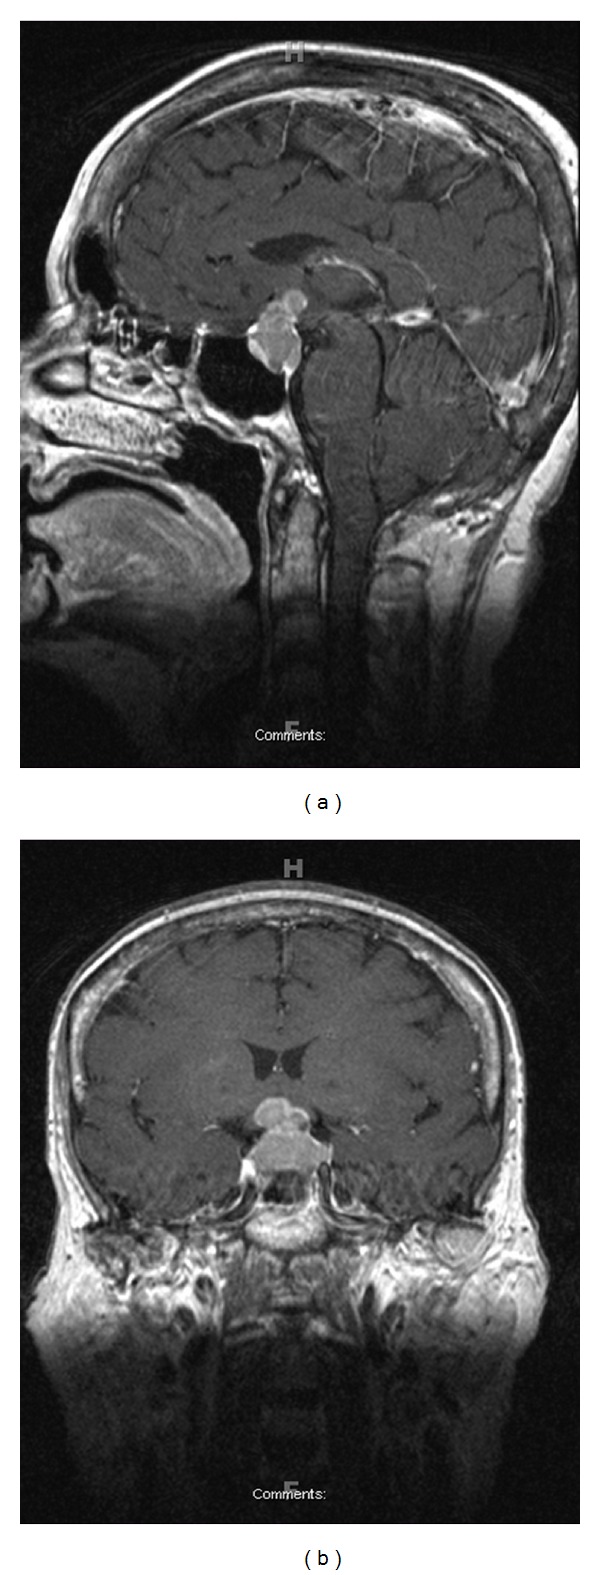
MRI brain, (a) coronal, (b) sagital: 2.0 × 1.2 × 1.1 cm bilobed homogeneous enhancing mass expanding the sella with compression of the optic chiasm.
